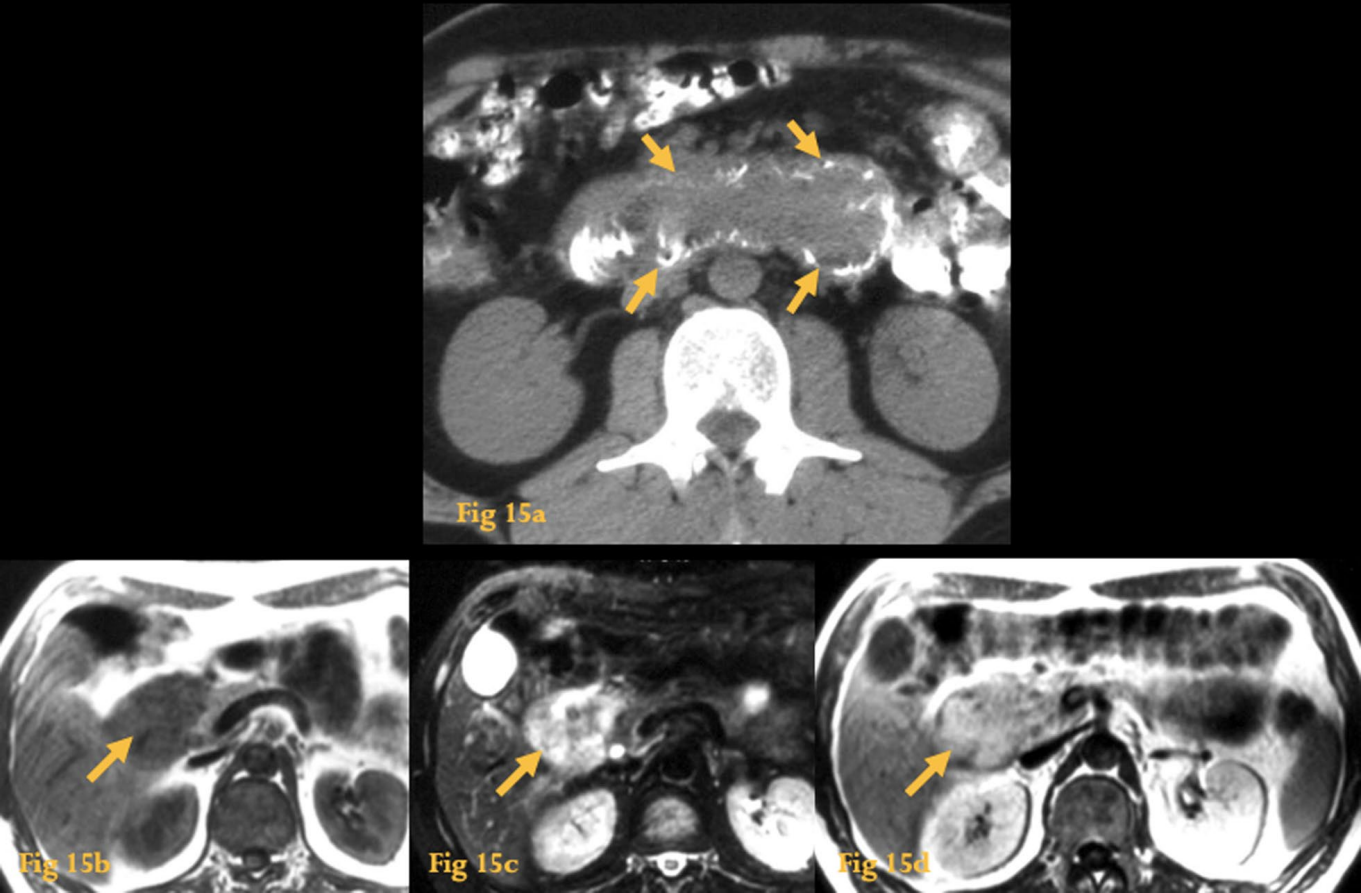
Hamartoma of Brunner’s glands. Unenhanced CT (a) shows a voluminous polypoid lesion originating in the duodenal bulb and reaching D4, with soft-tissue density and a lobulated contour (arrows). MRI of the same patient shows that the lesion (arrows) is heterogeneously hypointense on T1w images (b), hyperintense on FS T2w images (c), with enhancement after iv administration of gadolinium (d)
